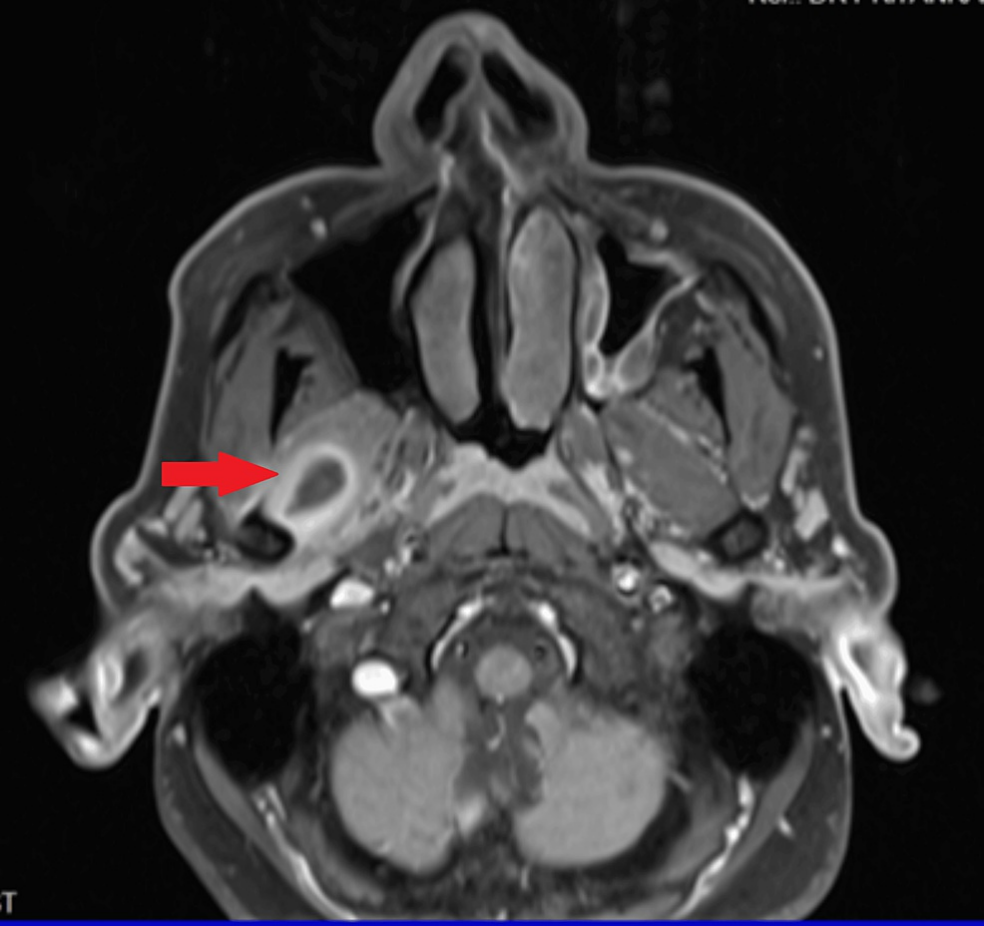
The axial section of the MRI shows a ring-enhancing lesion within the left lateral pterygoid muscle, suggestive of cysticercosis.
